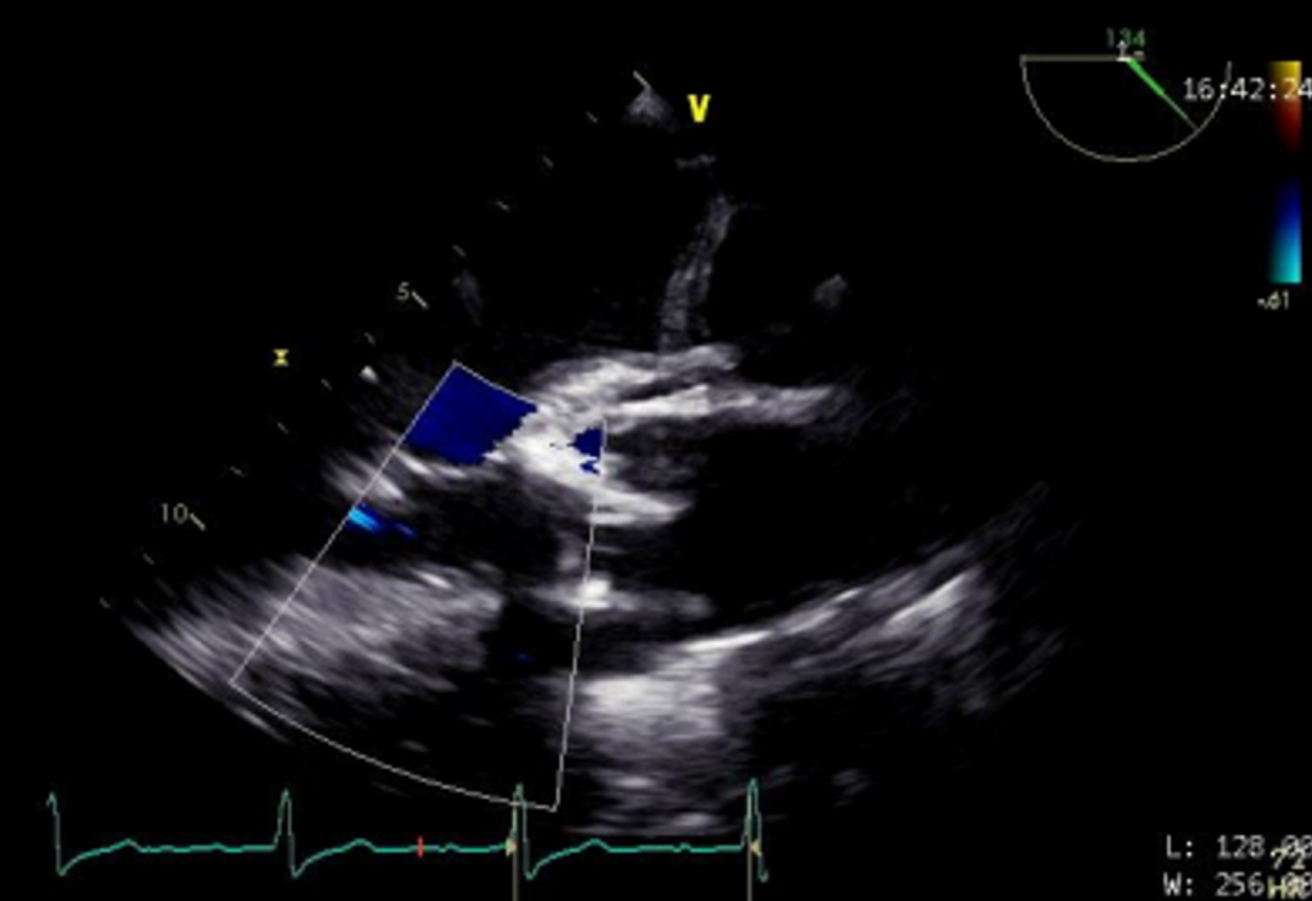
Aortic valve status post repair. TEE is vital after valve repair to assess for improved/unchanged/worsened valvular insufficiency which dictates any further surgical intervention. TEE, transesophageal echocardiography
